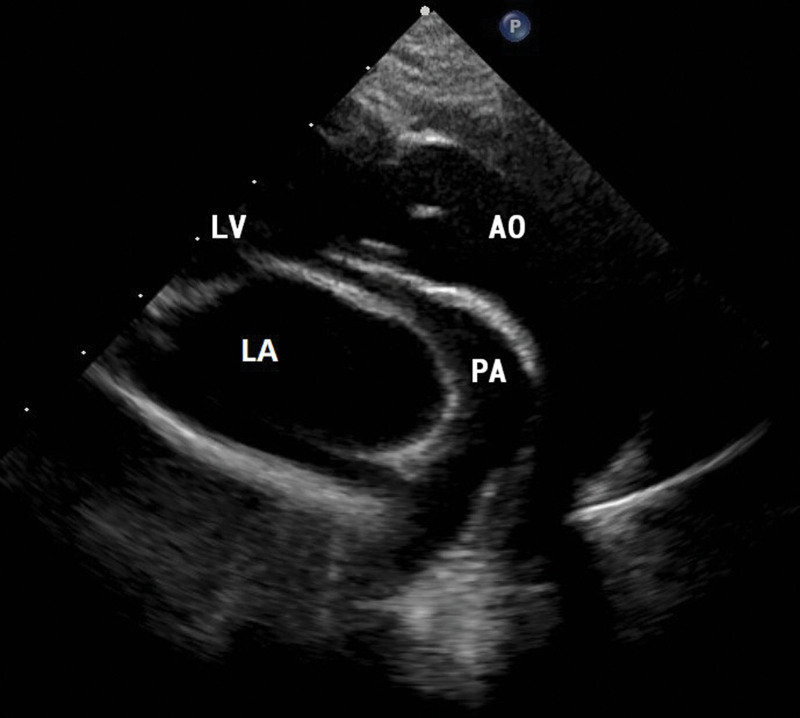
Transthoracic echocardiography (TTE) shows that the both great artery originated from LV, LV = left ventricle.
